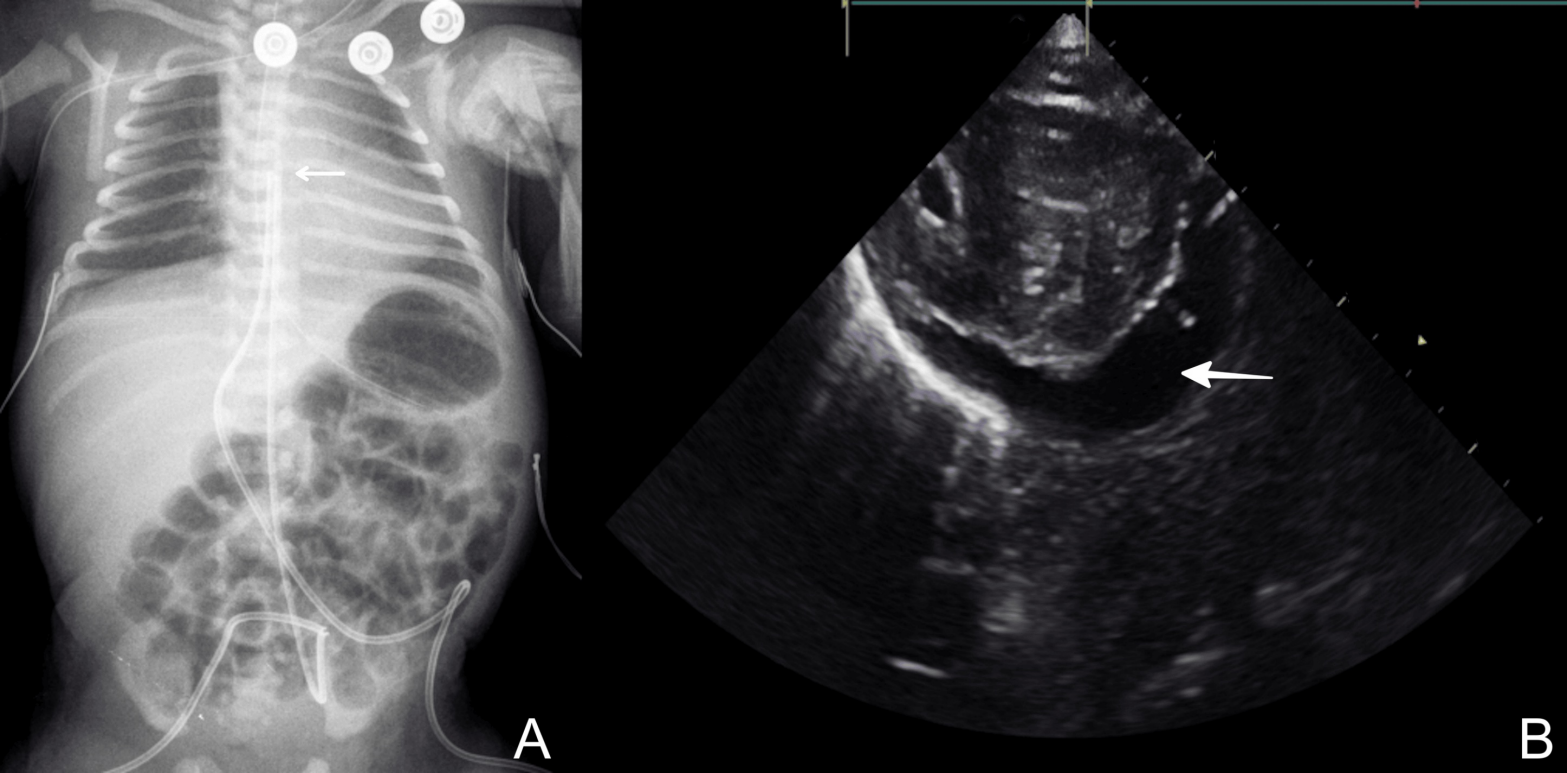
Case 2: (A) X-ray showing a high position of UVC (arrow). (B) Echocardiography showing the pericardial effusion (bold arrow). UVC: umbilical venous catheter
